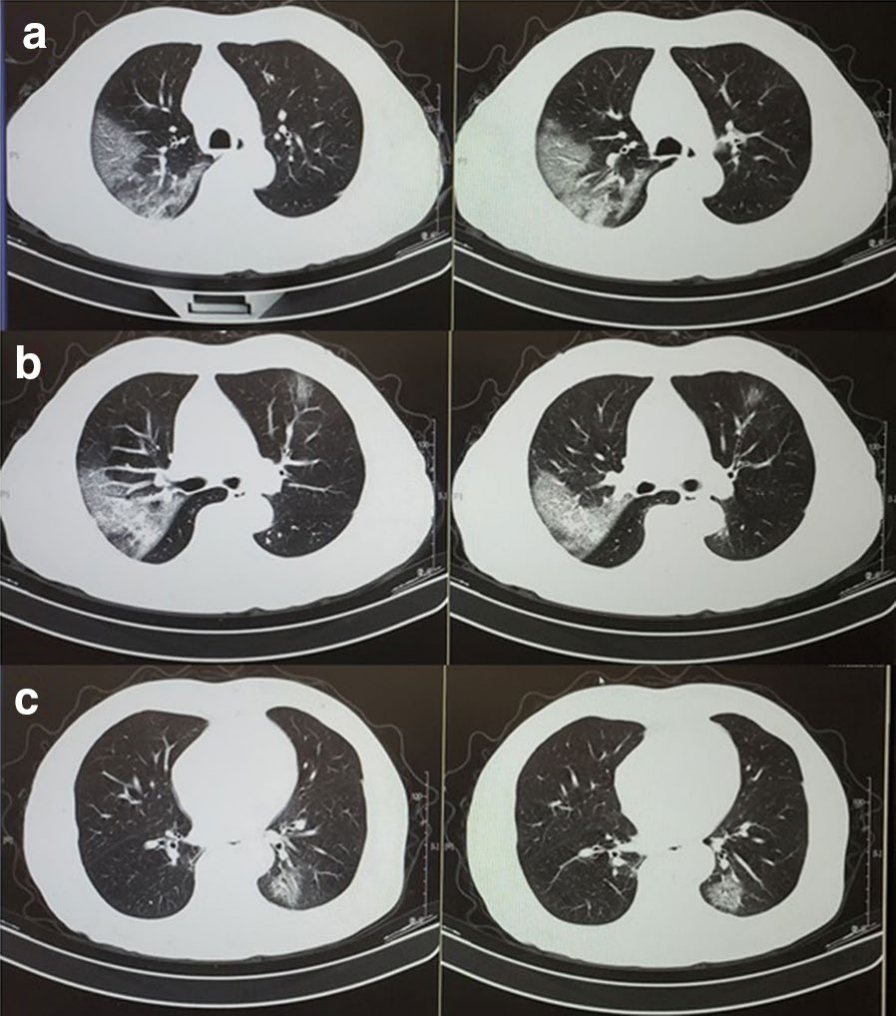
The multifocal ground glass changes on chest CT scan were typical of viral pneumonia in one infected 2019-nCoV patients. a shadow in right upper lobe; b shadow in bilateral lobe; c shadow in left lower lobe
